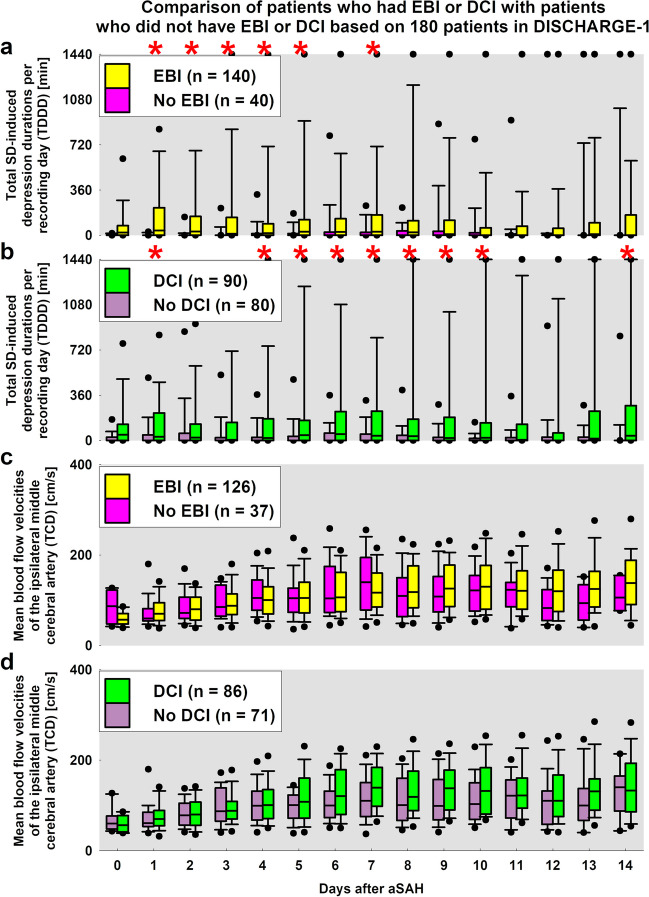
For each recording day, the respective total duration of activity depression induced by spreading depolarization (SD) of a recording day (TDDD) was compared between a patients with focal brain damage due to early brain injury (EBI) on the one hand and patients without focal brain damage due to EBI on the other hand and between b patients with focal brain damage due to delayed cerebral ischemia (DCI) on the one hand and patients without focal brain damage due to DCI on the other hand using Mann–Whitney rank sum tests and post-hoc Bonferroni correction. EBI was composed of focal brain damage due to intracerebral hemorrhage and early cerebral ischemia. Red asterisks indicate significant results after strict Bonferroni correction. c The comparison of mean blood flow velocities in the middle cerebral artery (MCA) ipsilateral to the subdural recording strip, measured by transcranial Doppler sonography (TCD), between patients with focal brain damage due to EBI compared to patients without focal brain damage due to EBI. d The comparison of mean blood flow velocities in the MCA between patients with focal brain damage due to DCI compared to patients without focal brain damage due to DCI. After strict Bonferroni correction, we could no longer detect any significant differences between the two groups compared in c and d, respectively. However, without strict Bonferroni correction, the mean velocities on the 15 days (= 15 tests) in d showed statistical differences between *p* = 0.0500 and *p* = 0.0033 on each day from day 6 to day 10 (= 5 tests). With 15 tests, only one uncorrected significant result is to be expected by chance. Since we observed significance in 5 out of 15 tests and then also on consecutive days, and considering that the statistical hypothesis tested is related to the same basic hypothesis—mean velocities correlate with delayed infarcts—one could argue that the Bonferroni correction is too conservative in this case. However, even without strict Bonferroni correction, it remains the case that the association of delayed infarcts with ECoG-measured TDDDs was stronger than their association with TCD-measured mean blood flow velocities
